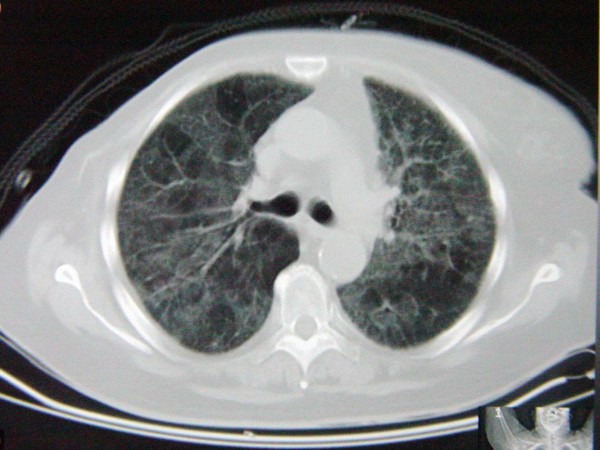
Computed tomography demonstrating patchy areas of ground-glass opacity.
